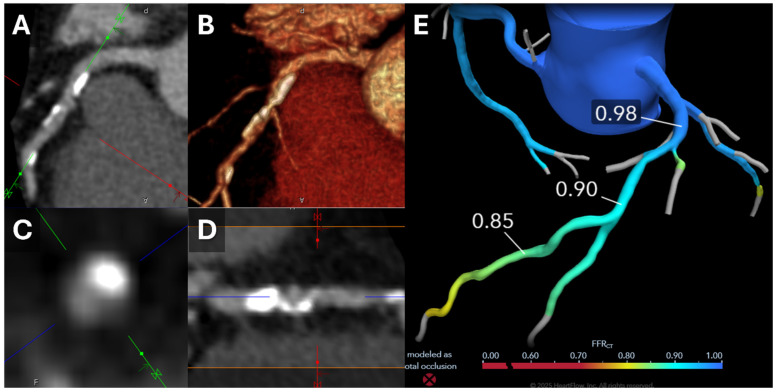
Use of FFRct. 54-year-old man presented to the ED with atypical chest pain; a contrast-enhanced coronary artery CTA was ordered to “rule out coronary artery disease”. Curved centerline 2D (A) and 3D (B) reconstructions through the proximal and mid-LAD from the CCTA demonstrate partially calcified atherosclerotic plaque. Short-axis (C) and straight center line (D) reconstructions through the mid-LAD demonstrate a focus of moderate (50–69%) stenosis. The study was sent for FFRct to determine whether the stenosis is hemodynamically significant. FFRct results (E) demonstrate a drop in FFRct values from 0.98 to 0.90 across the lesion, indicating a low probability that the stenosis is hemodynamically significant. The patient, based on these results, was medically managed rather than revascularized. The main advantage of FFRct is the ability to predict whether more borderline lesions in the 40–90% range are hemodynamically significant or not. Abbreviations: CCTA = coronary computed tomography angiography, FFRct = fractional flow reserve derived from computed tomography, LAD = left anterior descending artery.
